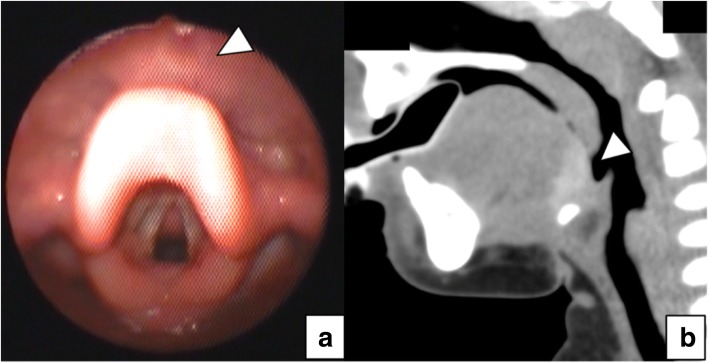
Postoperative imaging tests. Laryngeal endoscopy performed 6 months postoperatively (a) revealed the disappearance of airway compression by the lingual mass, and CT performed 8 months postoperatively (b) revealed relocation of the lingual thyroid gland towards the hyoid bone
